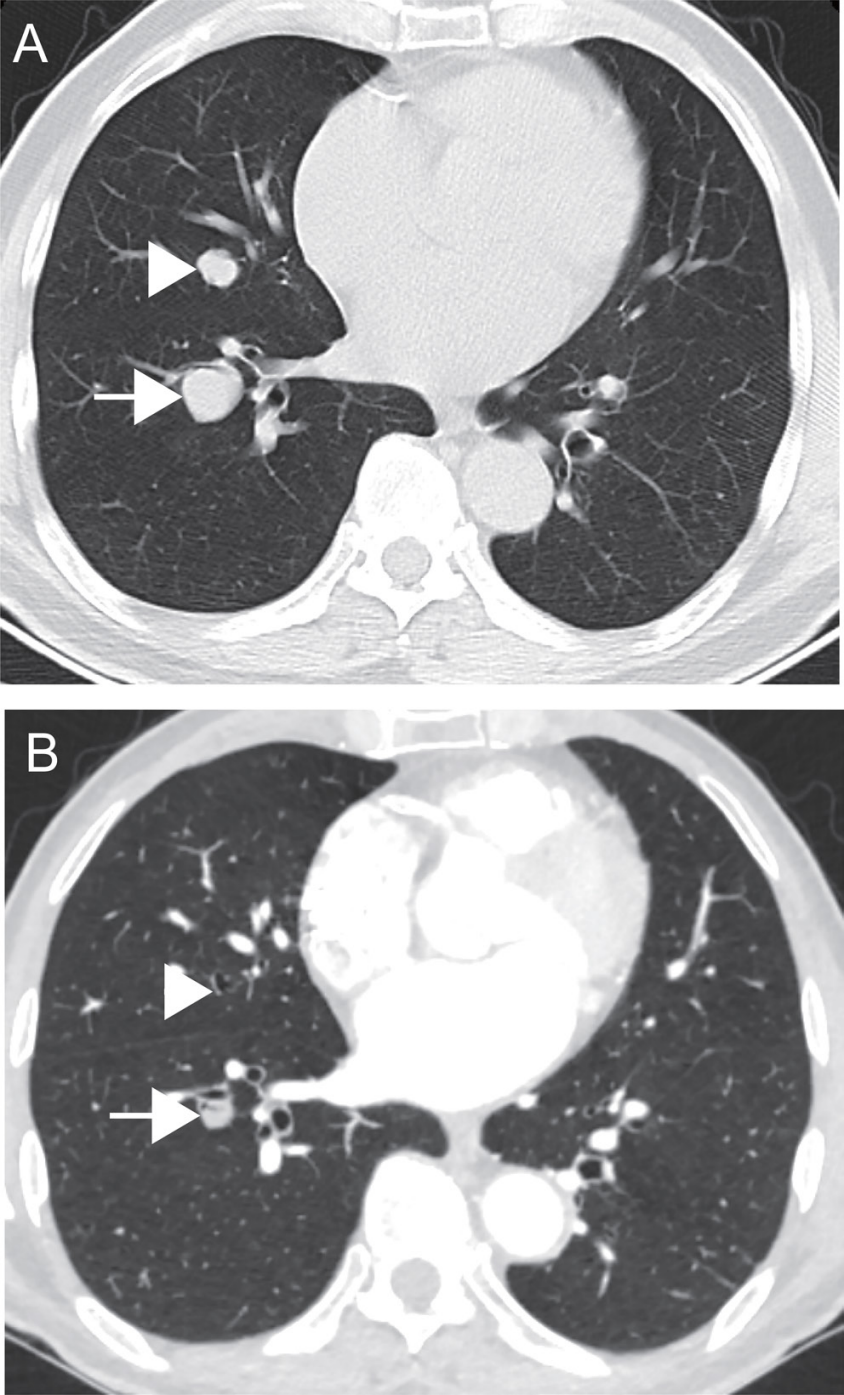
Notable shrinkage of a sorafenib-resistant radioiodine-refractory lung metastatic papillary thyroid cancer in a 62-year-old male patient treated with apatinib. (A) CT planar scan before apatinib treatment; (B) CT planar scan 5 months after apatinib initiation, showing a 67% decrease in the sum of the target lesion. Simultaneously, the serum thyroglobulin level dramatically declined from 938 to 38 ng/mL.
